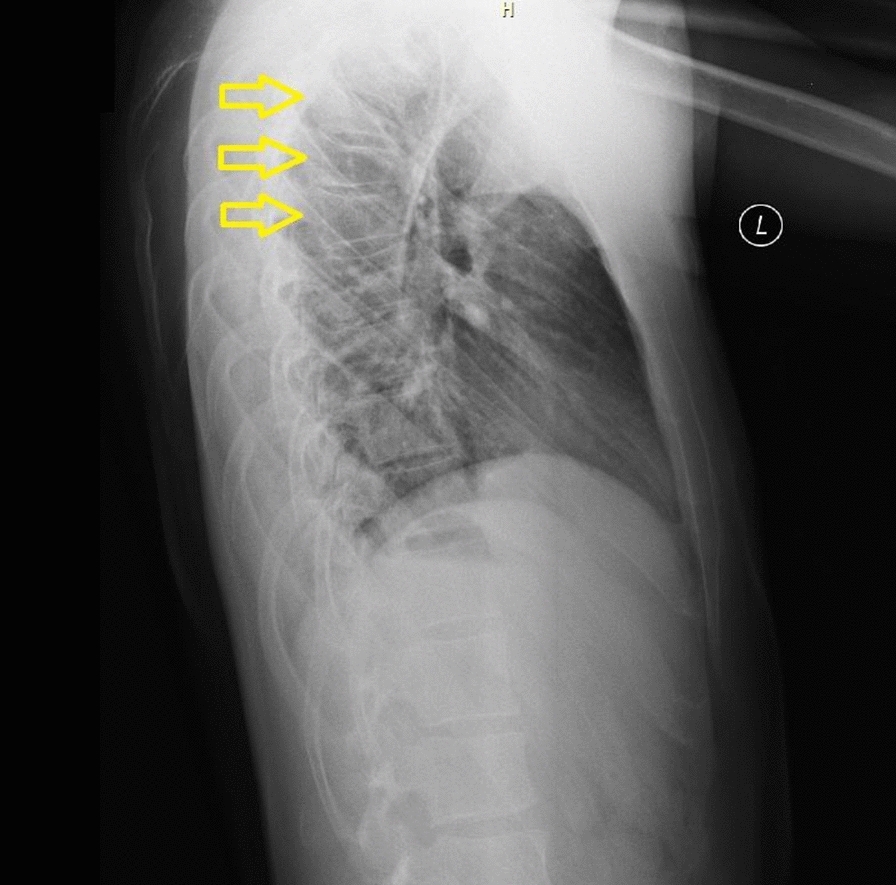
X-ray of thoracic vertebrae revealing Th3–5 compression fractures (yellow arrows)
